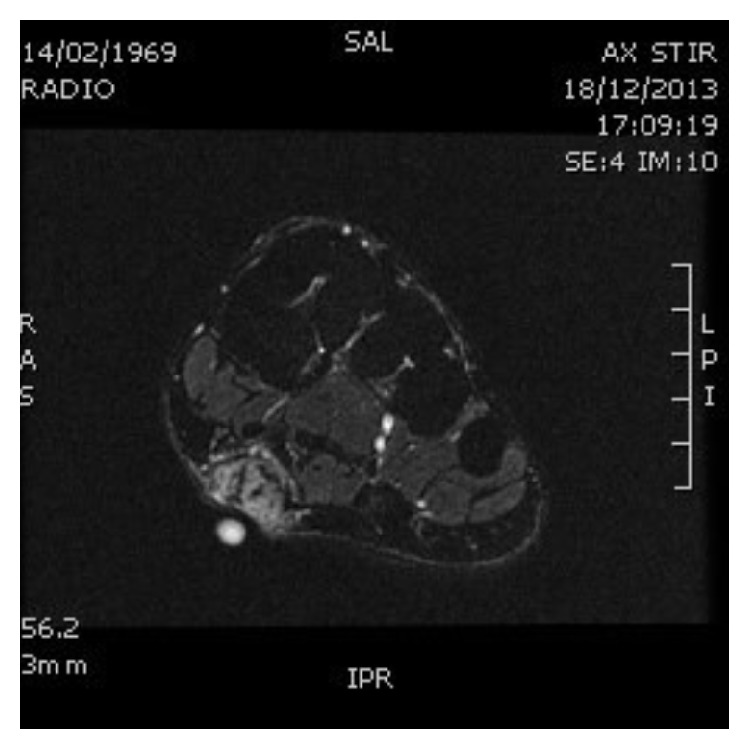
MRI of the right feet showing a nodule hyperintense in weight after saturation proton density fat signal.
